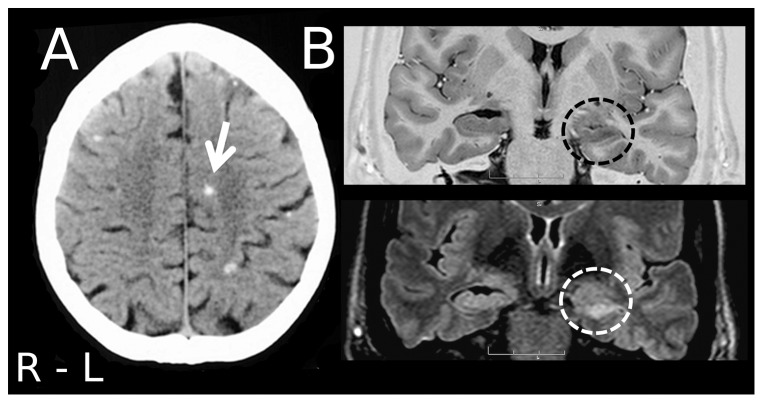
Neuroimaging of a patient with epilepsy with both, neurocysticercosis and hippocampal sclerosis. (A) A CT-scan shows calcified lesions typical of neurocysticercosis (arrow). (B) MRI of this patient shows abnormalities typical of mesial temporal lobe epilepsy associated with hippocampal sclerosis (circles).
